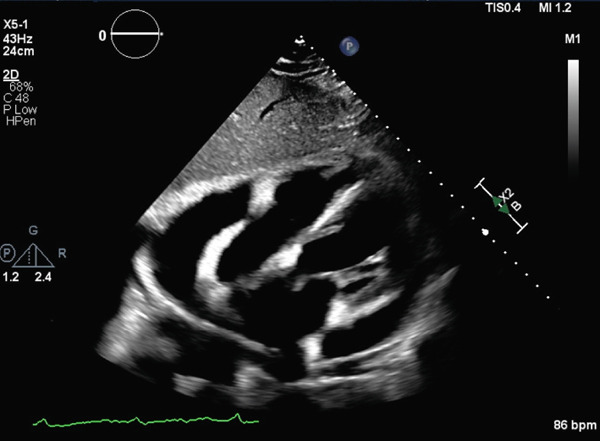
Echocardiographic image showing a large circumferential pericardial effusion with early tamponade physiology (right atrial diastolic collapse).
